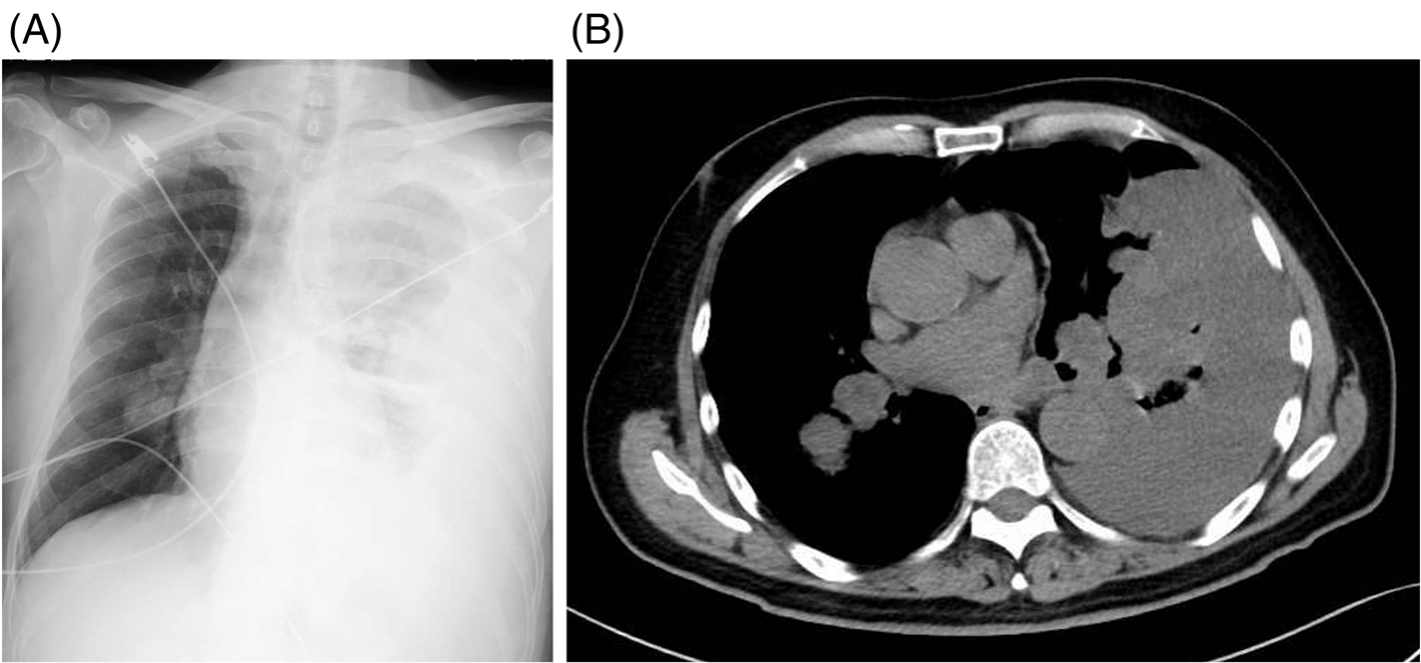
(A) Chest radiograph after emergency transport. (B) Plain CT image of the chest after emergency transport showing masses in both lung fields, left pleural effusion, and mediastinum deviated to the right
